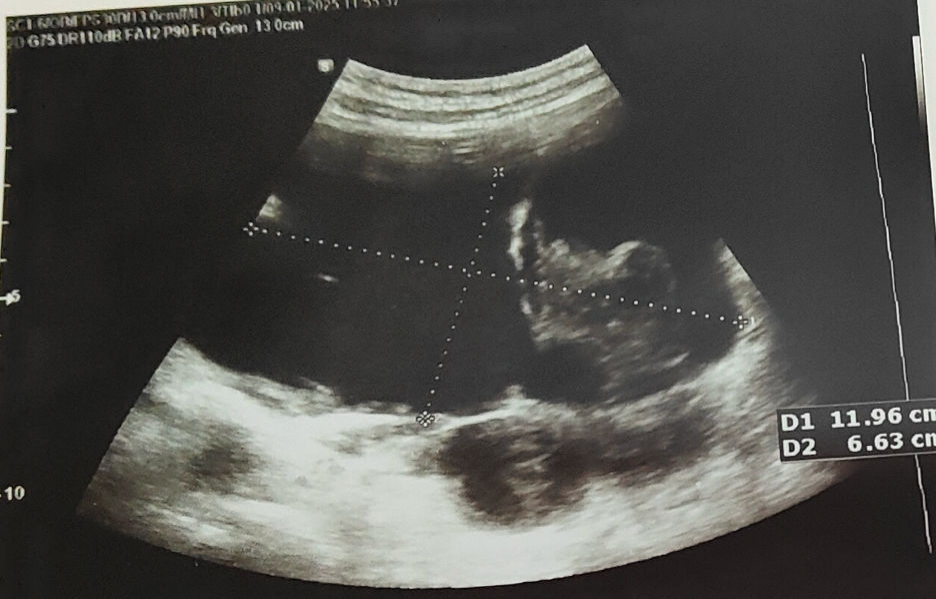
Ultrasound of the abdomen showed a mass of size 11.9 × 6.6 cm with multiloculated cyst having septations.
